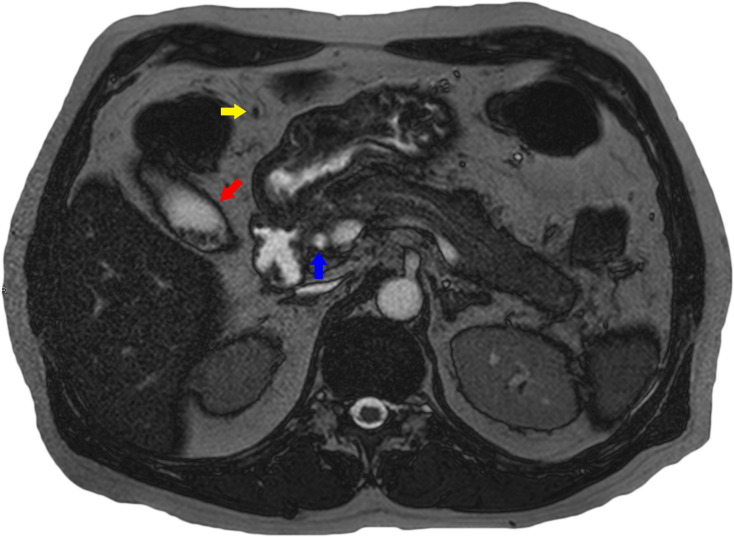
MRI transverse *T*_2_ weighted sequence demonstrating gallstones (red arrow), a normal calibre distal common bile duct with no internal filling defects/stones (blue arrow) and a normal non-dilated paraumbilical vein (yellow arrow).
